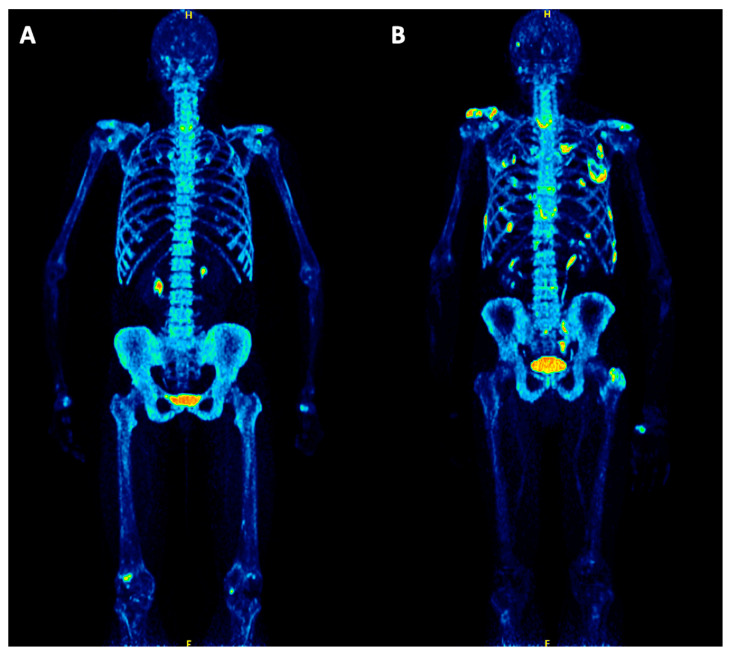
Maximum intensity projection (MIP) PET/CT images of two symptomatic multiple myeloma (MM) patients before treatment. (A) PET/CT of a 66-year-old female patient shows no ^18^F-NaF positive, skeletal myeloma lesions. However, several degenerative changes are depicted, for example in the spine, shoulders, hands and knees. (B) PET/CT of a 60-year-old male patient demonstrating multiple focal, ^18^F-NaF positive myeloma lesions in the scapula, humerus, spine, pelvis, femur and ribs, partially corresponding to pathologic rib fractures.
